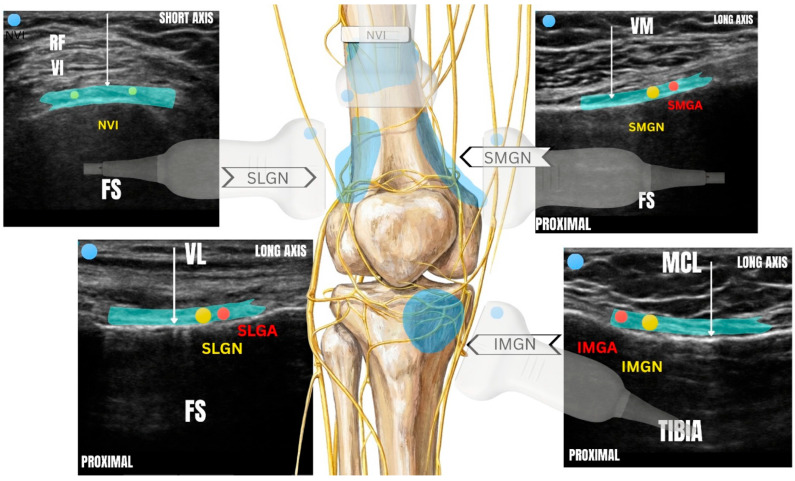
Anatomical representation and ultrasound-relevant anatomy for the four-nerve genicular block.
